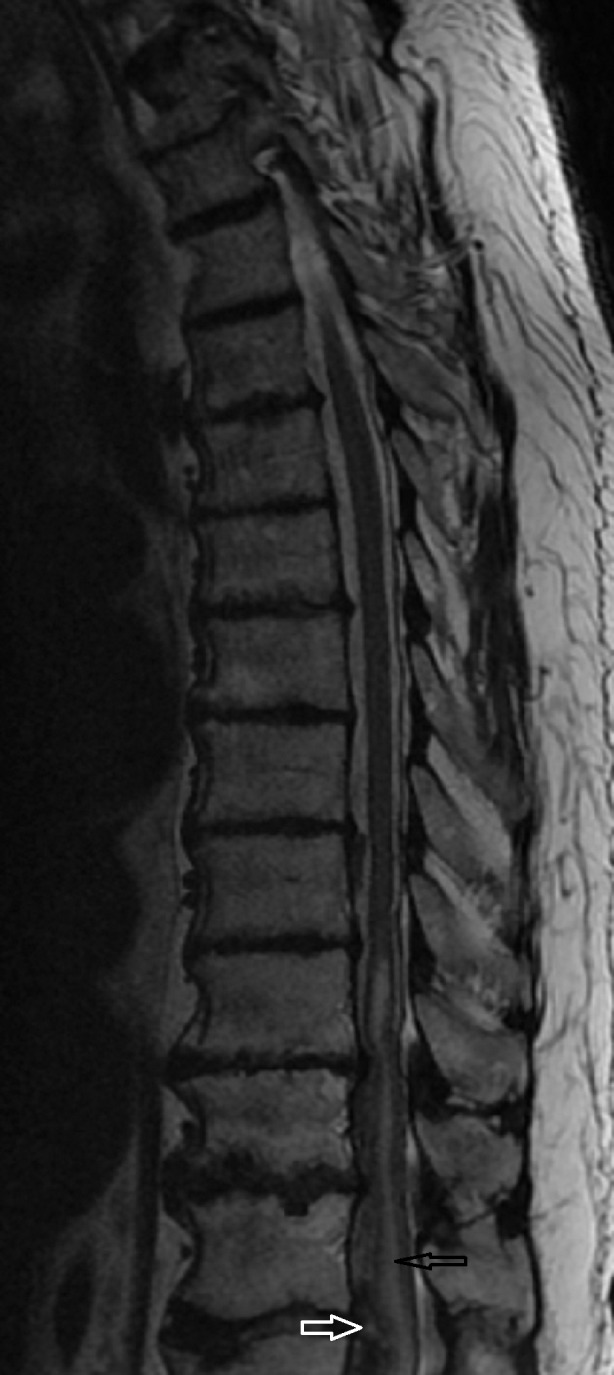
MRI Thoracic Spine T2 Weighted Black arrow shows hematoma. White arrow shows edema in the thoracic spinal cord.
